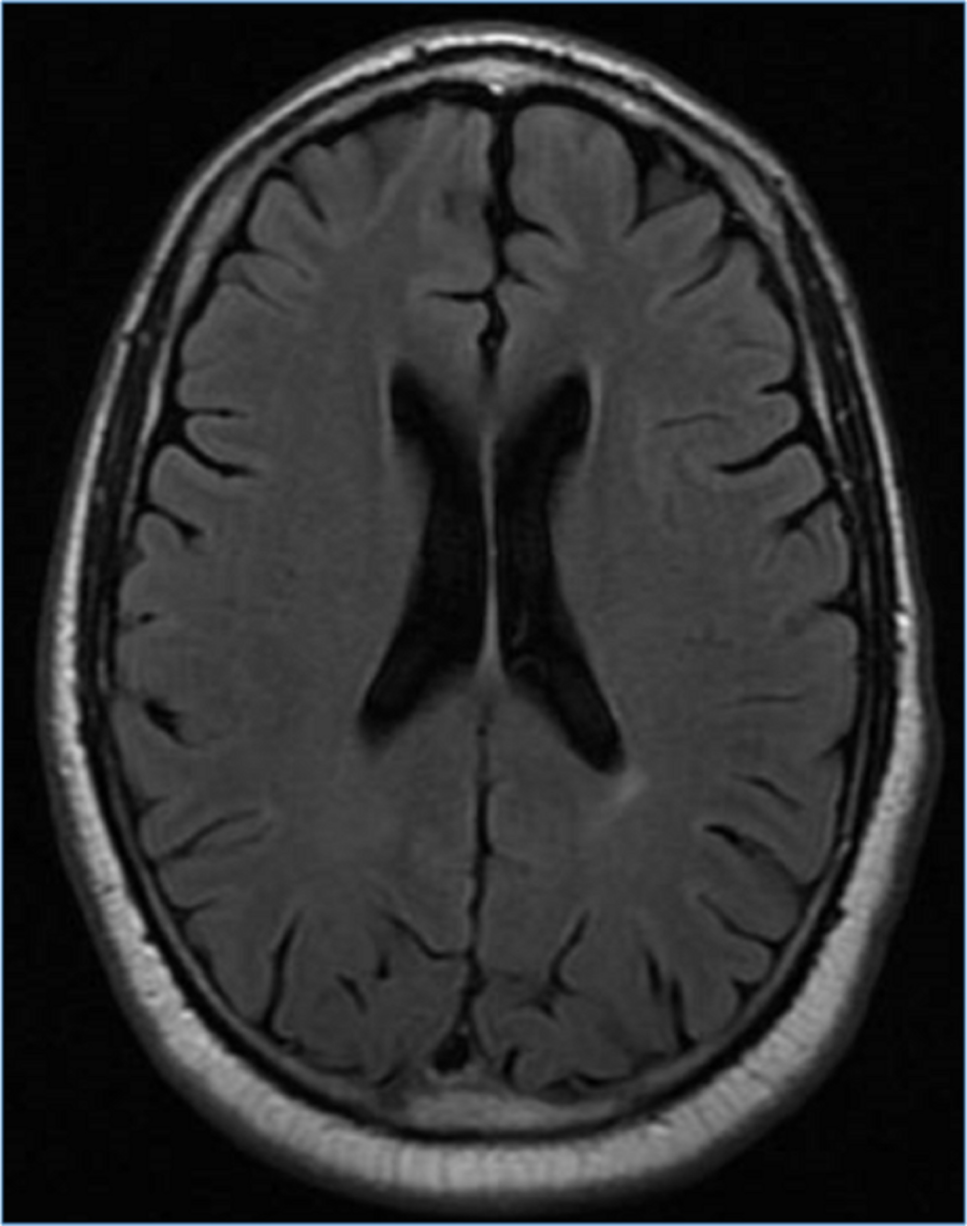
Magnetic resonance imaging (MRI) of the brain did not reveal any acute abnormality
